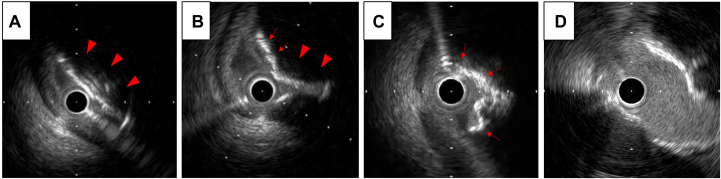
Intravascular Ultrasonography Images (A) Intravascular ultrasonography (IVUS) showed a nodular calcification on the upper side of the right coronary artery (RCA) ostium (arrowheads) and compression of the lumen by the plaque. (B) After the rotablation with a 2-mm burr, a considerable number of calcified nodules remained (arrowheads), although the lumen had widened partially (arrows). (C) After rotablation with a 2.25-mm burr, the calcified lesion in the RCA ostium was relatively evenly shaved (red arrows). (D) After the percutaneous coronary intervention, adequate dilation of the lesion was confirmed by IVUS.
